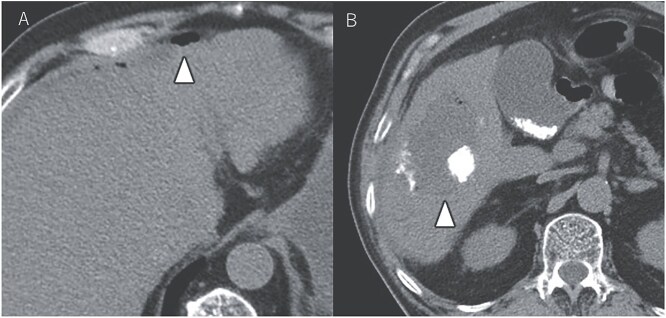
CT images showing tumor and enlarged lymph nodes in region 12b before ATZ + BV therapy (A and B). After chemotherapy, reduction of the tumor and lymph nodes is evident (C and D). Notably, no abscess formation was observed in the HCC or surrounding liver tissue, highlighting the direct communication between the tumor and the colon.
